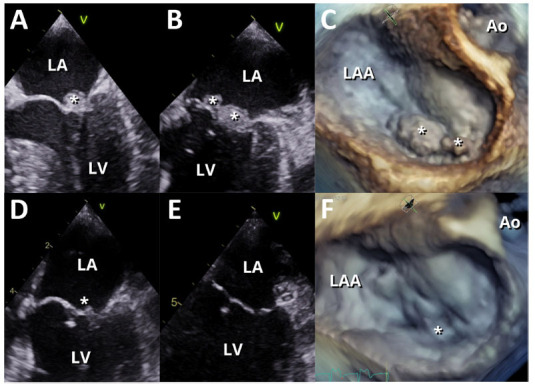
Baseline and follow-up 2D and 3D echocardiograms. Two-dimensional echocardiogram in the apical 4- (A) and 2-chamber (B) views, showing a vegetative lesion on the posterior cusps, P2 and P3. (C) Three-dimensional transesophageal echocardiogram (TEE) showing vegetative lesions on the posterior cusps in P2 and P3. Two-dimensional TEE after two weeks of subcutaneous low-molecular-weight heparin and one month of rivaroxaban treatment, demonstrating near-complete resolution of vegetations, in the apical 4- (D) and 2-chamber (E) views. (F) Three-dimensional TEE after two weeks of subcutaneous low-molecular-weight heparin and one month of rivaroxaban treatment, demonstrating near-complete resolution of vegetations. LA: left atrium; LAA: left atrial appendage; LV: left ventricle; Ao: aorta; (*): vegetations.
